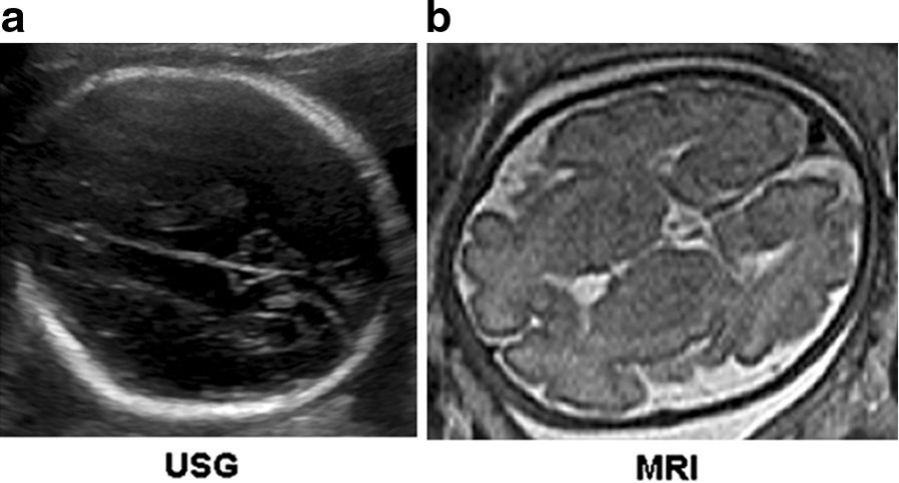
Ultrasonography (USG) vs. magnetic resonance imaging (MRI). Fetal MRI was used as a complimentary modality to USG. a USG. b MRI
